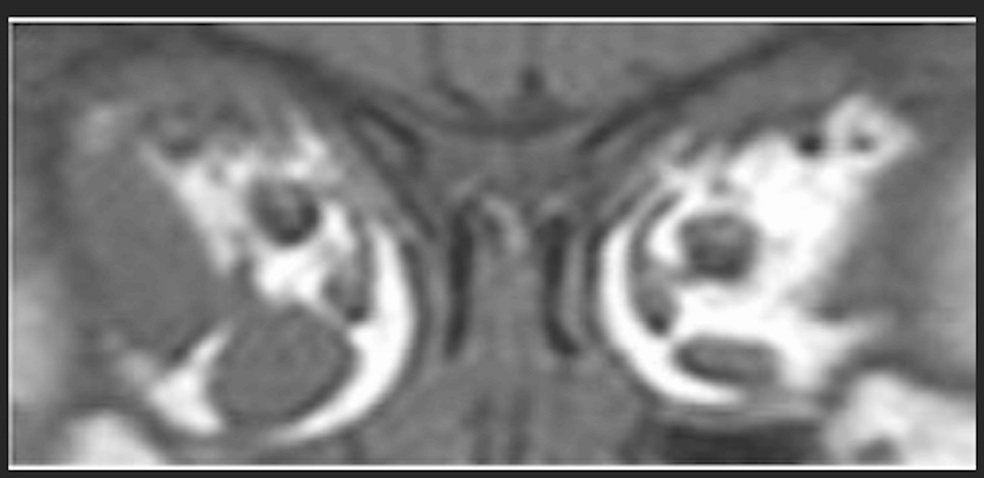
MRI findings one year after the second surgery. MRI findings were the same as the first MRI. MRI, magnetic resonance imaging
